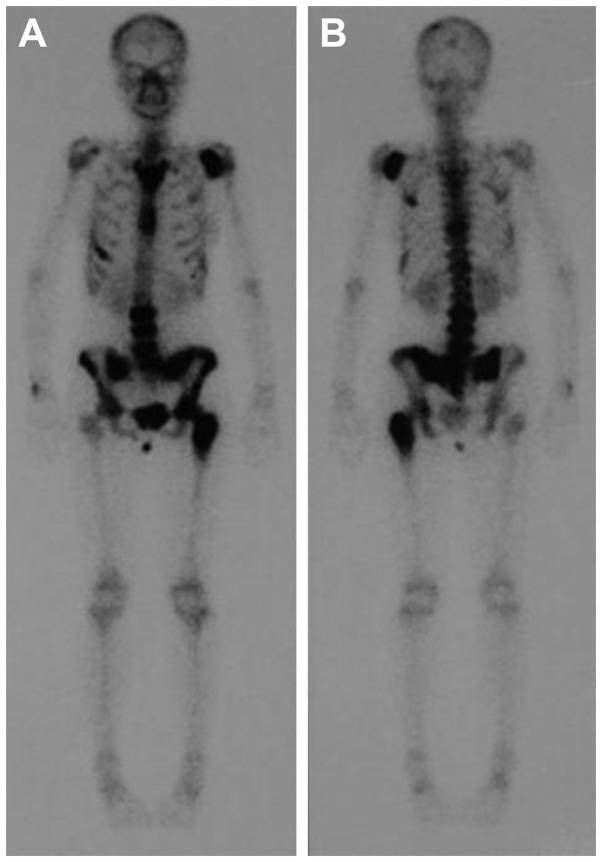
Single-photon emission computed tomography (CT) revealing abnormal bone metabolism of the lumbar, sacroiliac joint, the left greater trochanter and the left shoulder. CT of the lung and mediastinum reveals no abnormalities, except for sclerotin destruction in the left shoulder. (A) Front view and (B) rear view.
